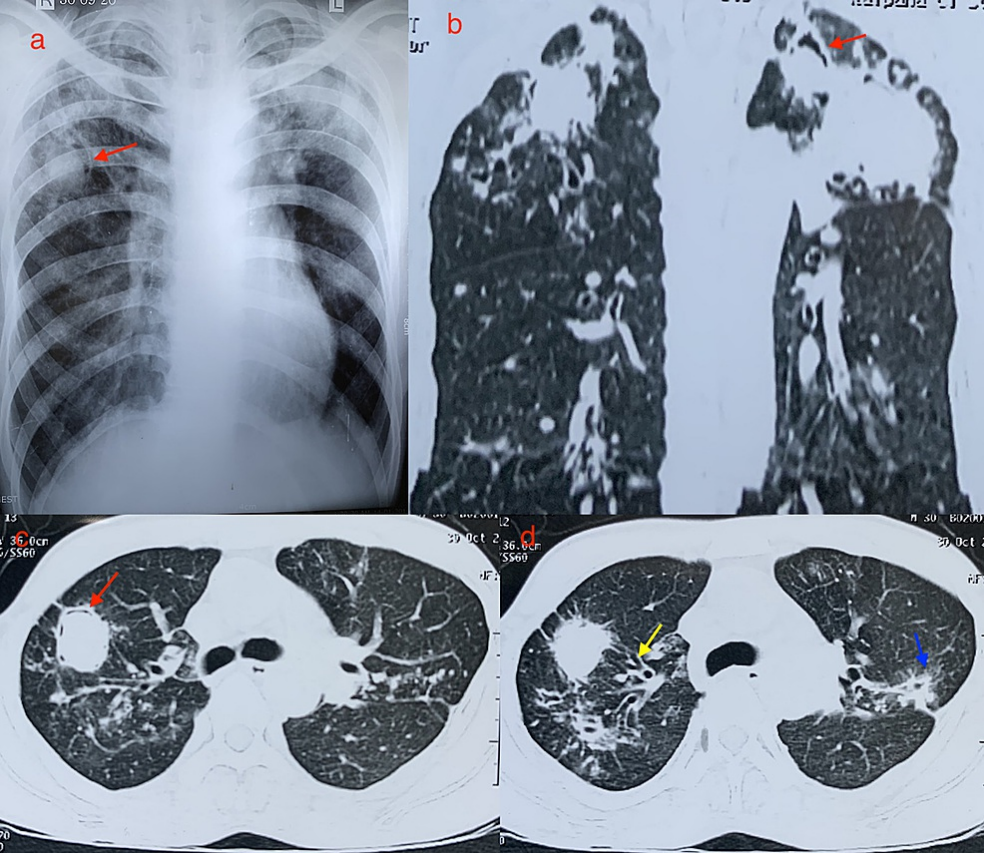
Chest X-ray and CT scan of the chest (sagittal and axial views). (a) Chest X-ray showing peripheral air crescent sign (red arrow). (b) Sagittal CT scan of the chest showing air crescent sign (red arrow). (c) Axial CT of the chest showing peripheral air crescent sign (red arrow). (d) Axial CT of the chest showing air crescent inside the nodule with a halo sign (blue arrow) and tree-in-bud (yellow arrow).
